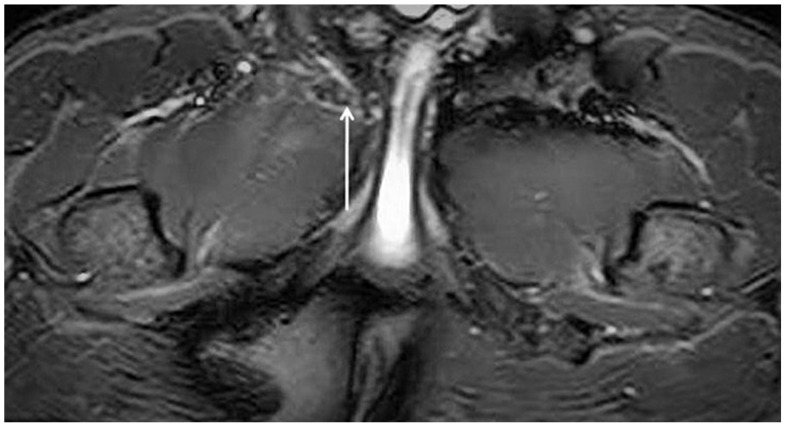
Axial oblique PD FS MRI showing a right adductor longus tendinopathy (arrow).
